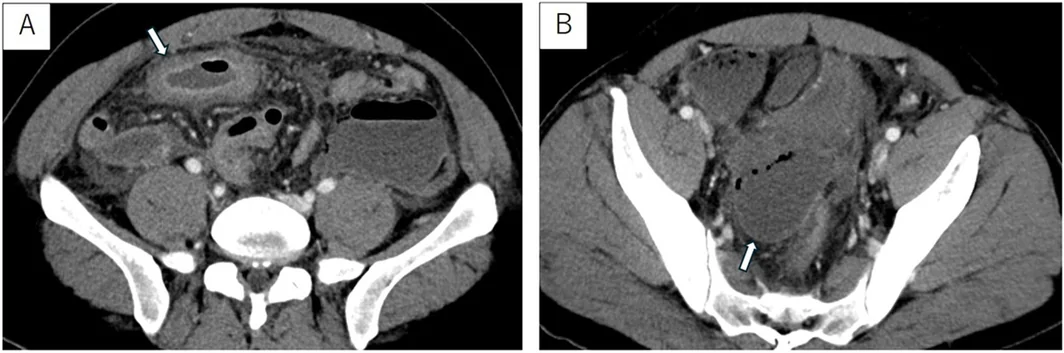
Contrast‐enhanced abdominal CT on the third day of admission showed free air in the abdominal cavity, increased fat density around the small intestine (A), and fluid accumulation in the abdominal cavity that indicated an intra‐abdominal abscess (B). Arrows indicate the affected small intestinal segment (A) and intra‐abdominal fluid collection (B).
